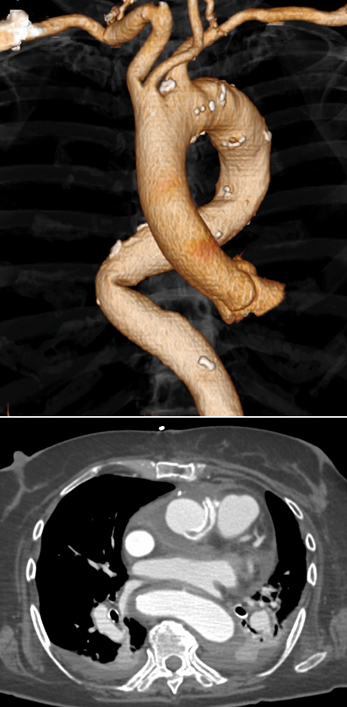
CT demonstrated a tortuous remodeled descending aorta with AEF after type A IMH surgery.
